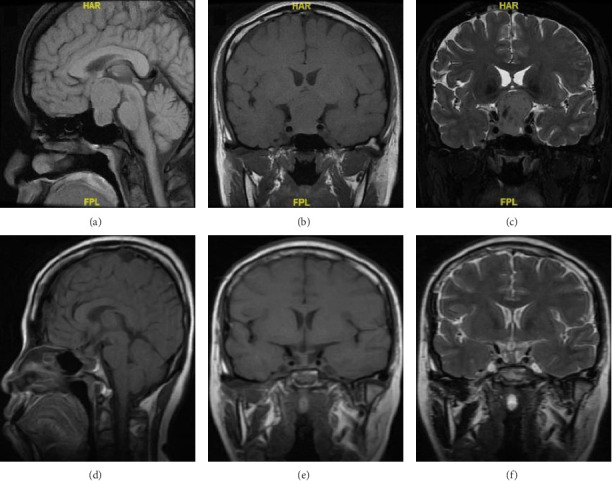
Figures (a and b) show preoperative T1 sagittal and coronal MRI images, respectively. Figures (d and e) show 16-month postoperative T1 sagittal and coronal MRI images, respectively. Figures (c and f) show preoperative and 16-month postoperative T2 coronal MRI images, respectively.
